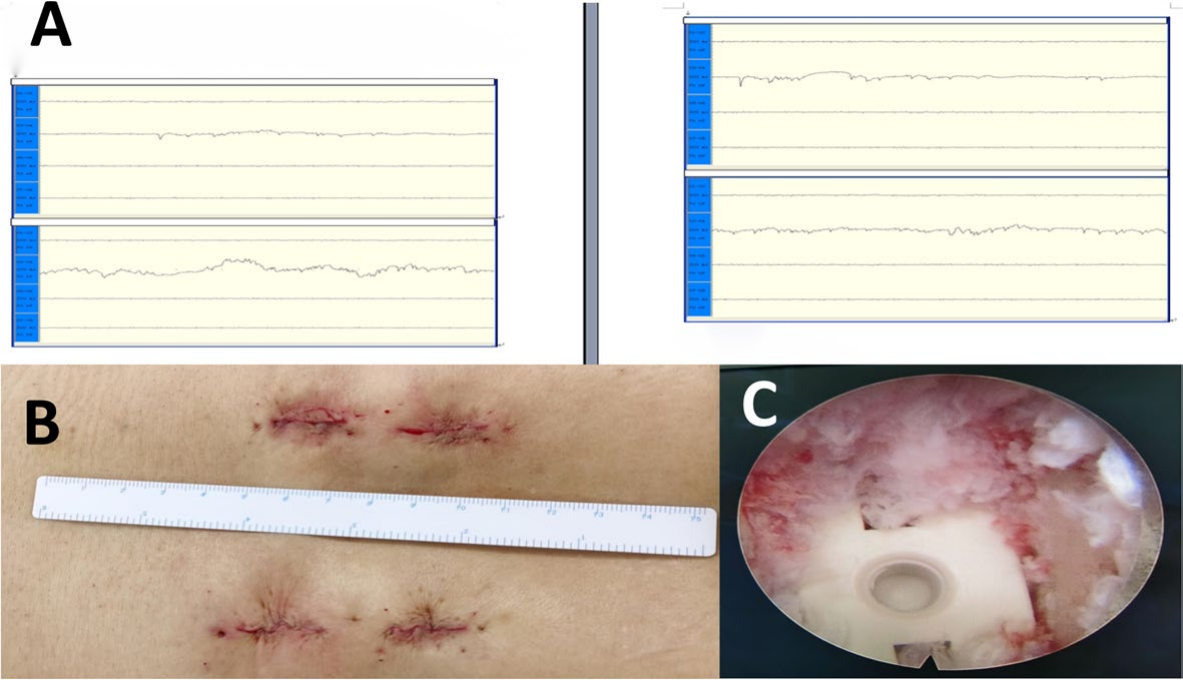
Postoperative radiographs (A and B) and CT scan (C)
